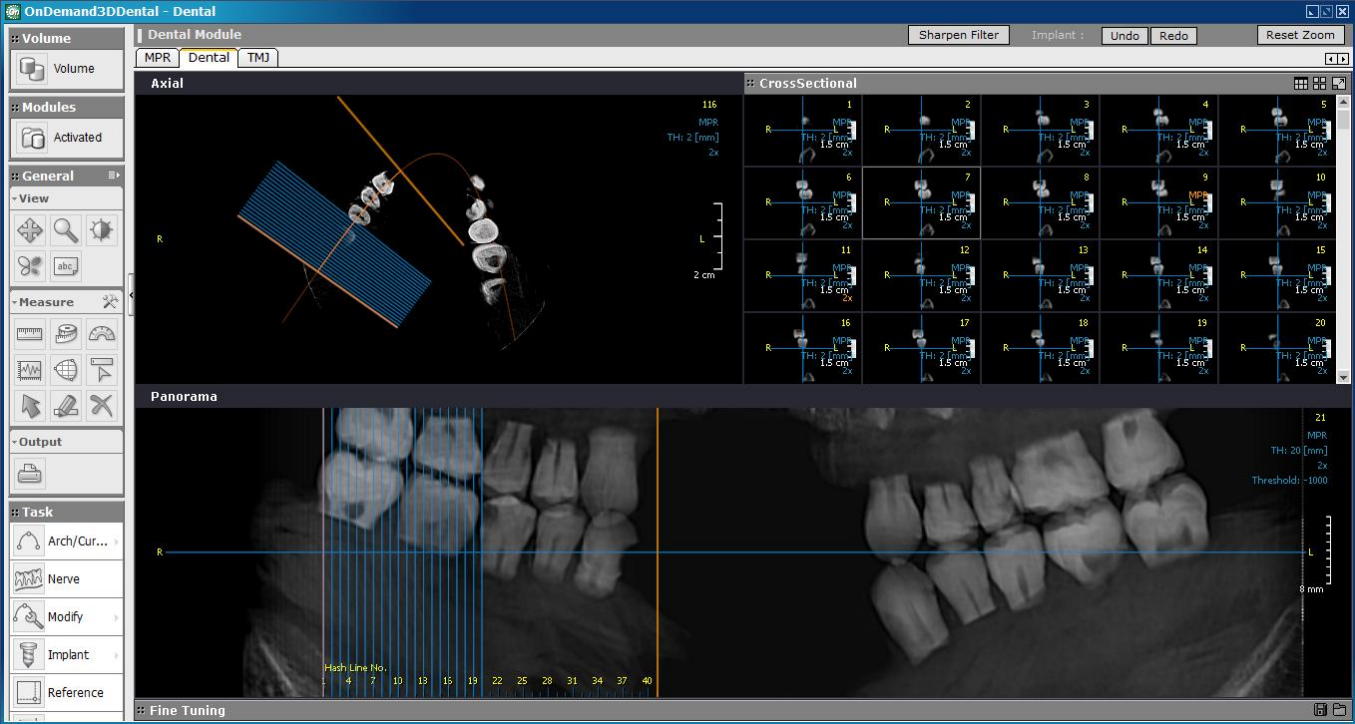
Cone-beam computed tomography (CBCT) image of the skull containing the teeth
